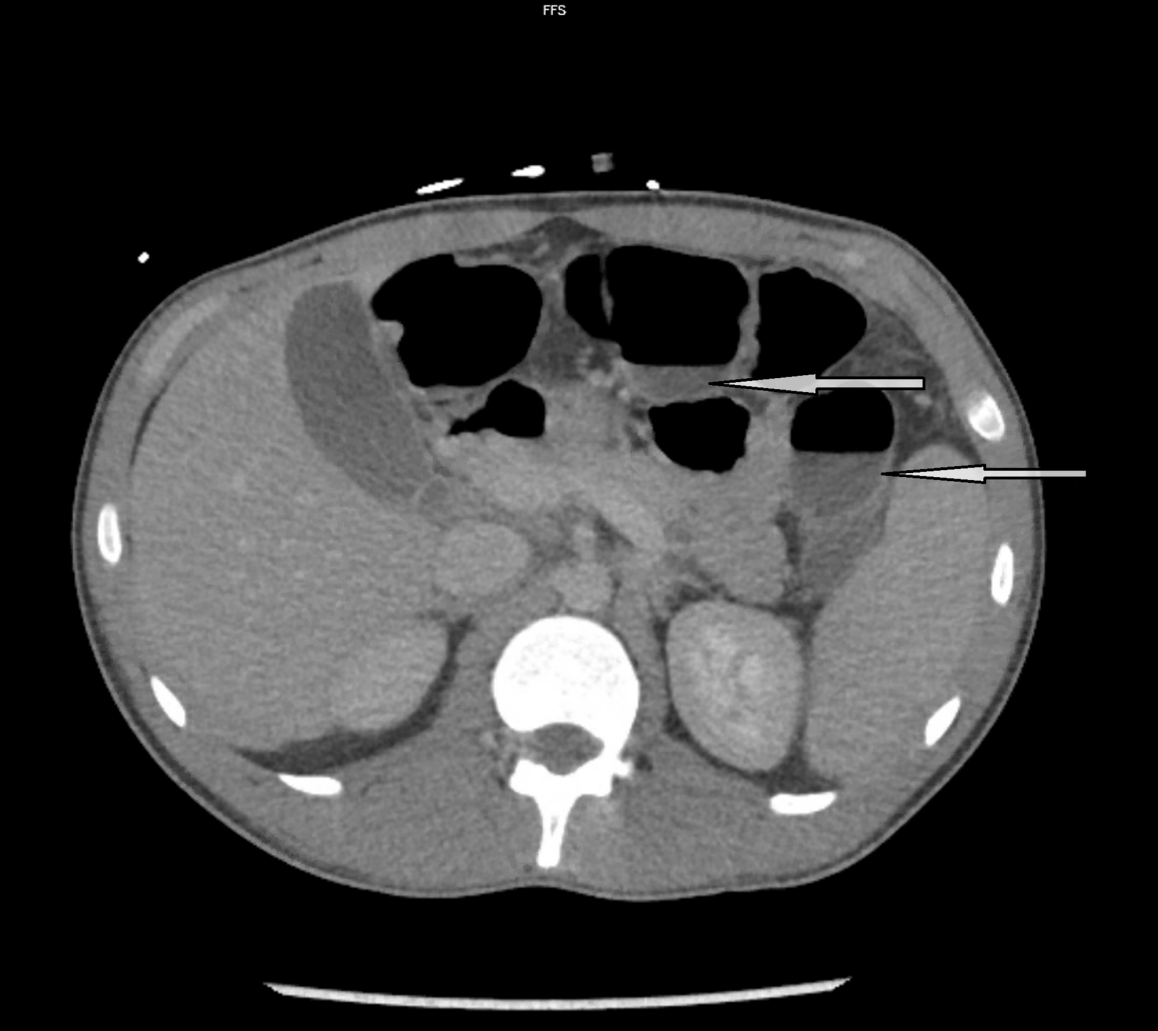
CT scan of the abdomen shows fluid-filled bowels (pointing arrows)
